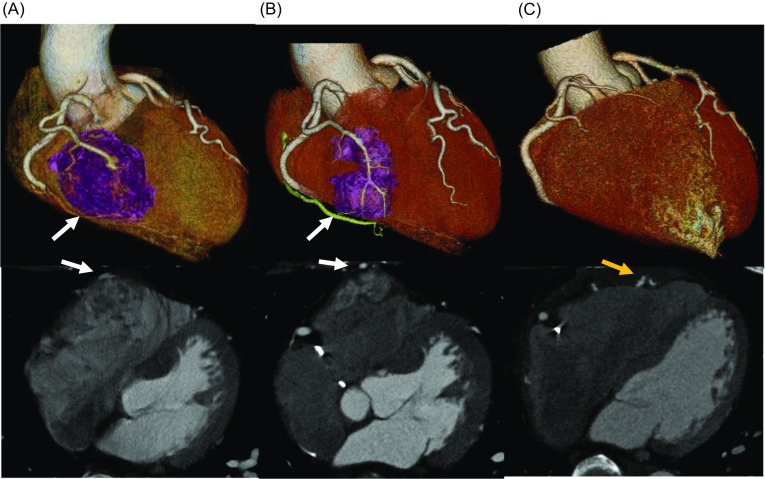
Enhanced three-dimensional computed tomography showing the RV metastasis due to renal cell carcinoma before the first operation (A), the recurrence of the RV (B) (each purple area), and the complete removal of the RV tumor after reoperation (C). White arrows show the RV branch of the right coronary artery. A yellow arrow shows the Hemashield Woven Double Velour Cardiovascular Fabric^®^. *RV* right ventricular
